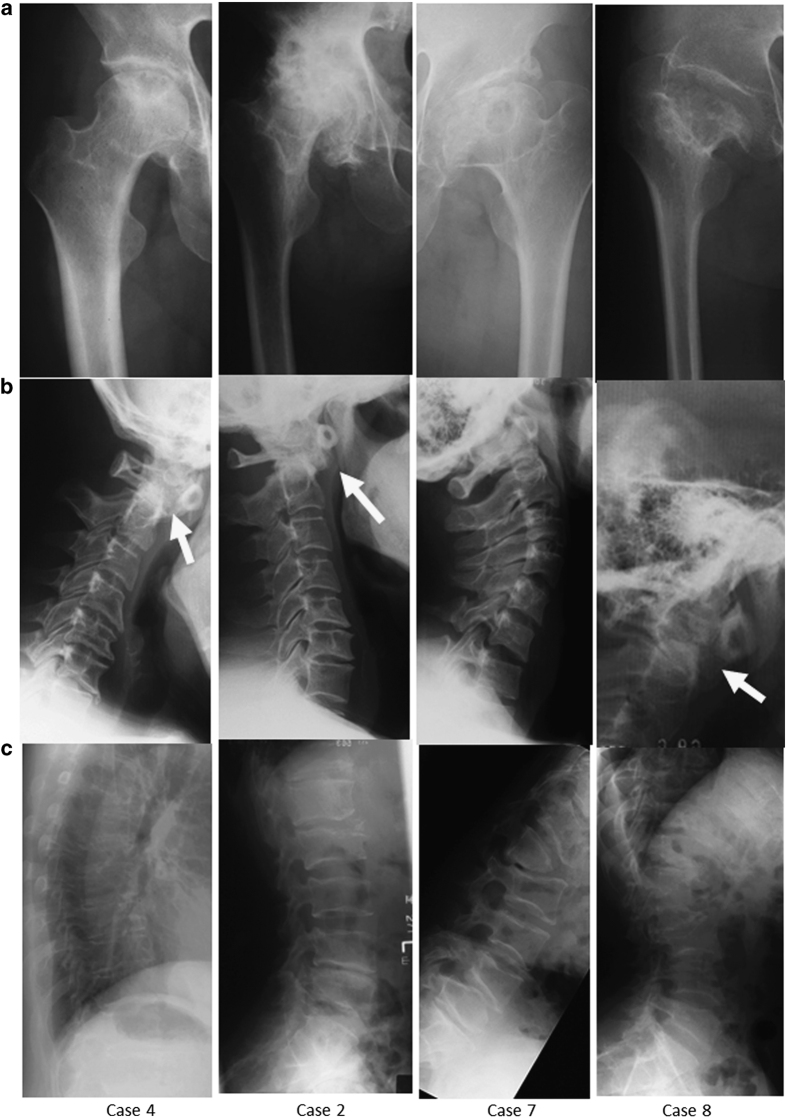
Radiographic findings of the family. (a) Hip joint: cystic and sclerotic changes in right femoral heads and the joint spaces were narrowed in case 4. Although structural deformation such as acetabular dysplasia was not common, joint spaces were narrowed in cases 2 and 7. In case 8, significant deformations such as high landing greater trochanter and coxa plana were confirmed. (b) Cervical spine: atlantoaxial subluxation with hypoplasia of odontoid process was confirmed in cases 4, 2 and 8, which was not confirmed in case 7. (c) Thracolumbar spine: although there are differences in the severity, platyspondyly was confirmed in all cases. In cases 7 and 8 with significant deformation, hypoplasia of the vertebral body and various slippages were confirmed.
